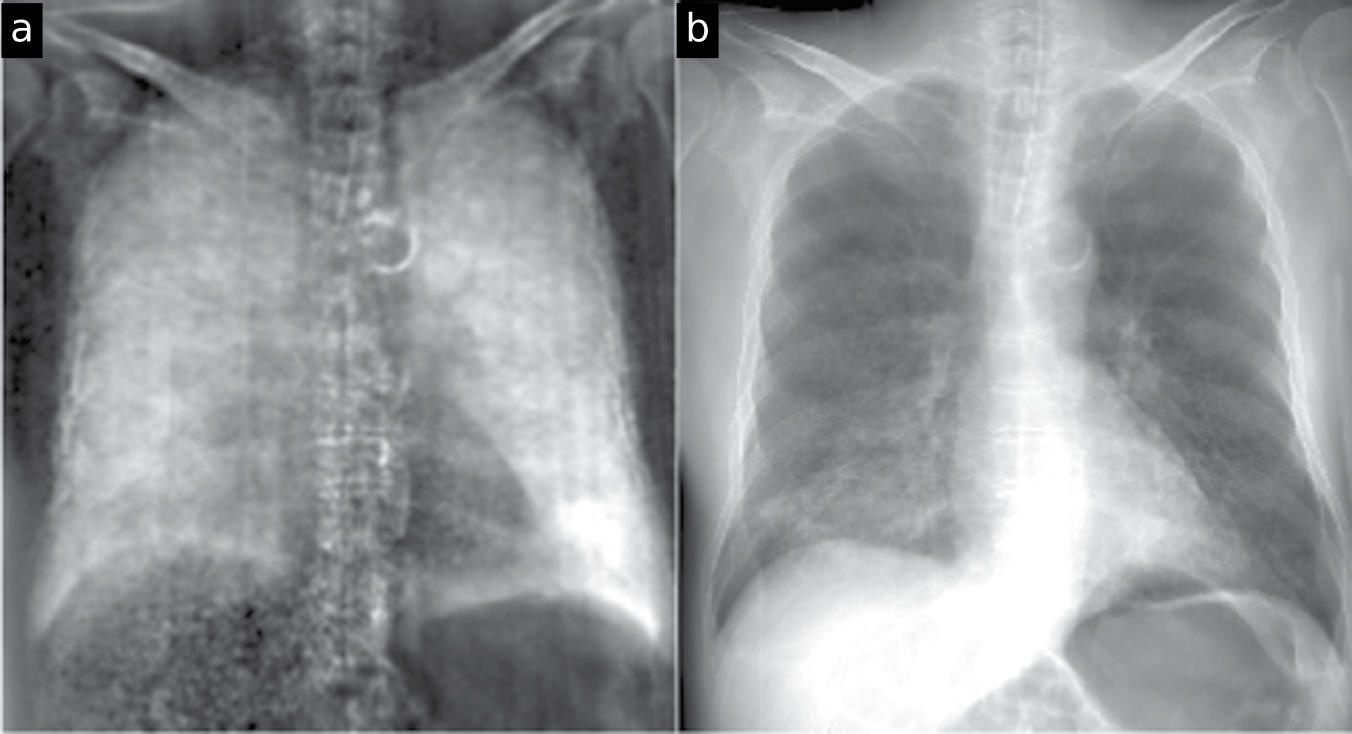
X-ray dark-field (a) and transmission (b) radiograph of a human thorax at 60kVp. The dark-field image was low-pass filtered by convolution with a 2D Gaussian kernel (*σ* = 3.2 px). In the transmission image, an infiltrate is visible in the lower left lung, which translates to a lower dark-field signal in this zone. Of all examined tube voltages (see also Fig. 2), the highest dark-field signal strength can be found at 60 kVp.
